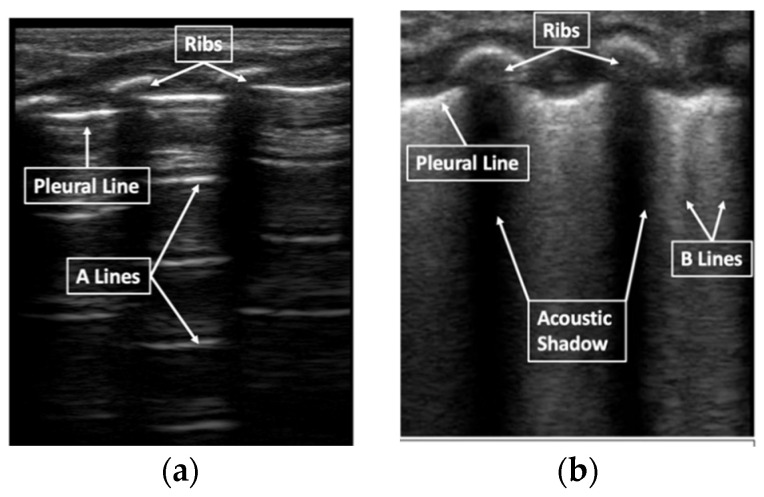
Lung ultrasound artifacts. B-model normal lung (a) B-model respiratory distress syndrome (b).
